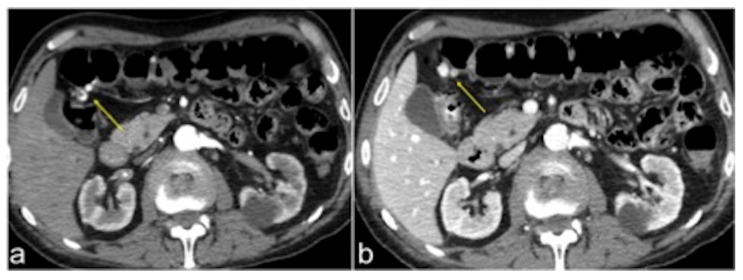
CTA multiphasic study in the arterial (a) and venous (b) phases of diverticular bleeding; a haemorrhagic spot in a diverticular sac of the colon at hepatic flexure is visible in the arterial phase (a, arrow) with increase and intensification in the venous phase (b, arrow).
